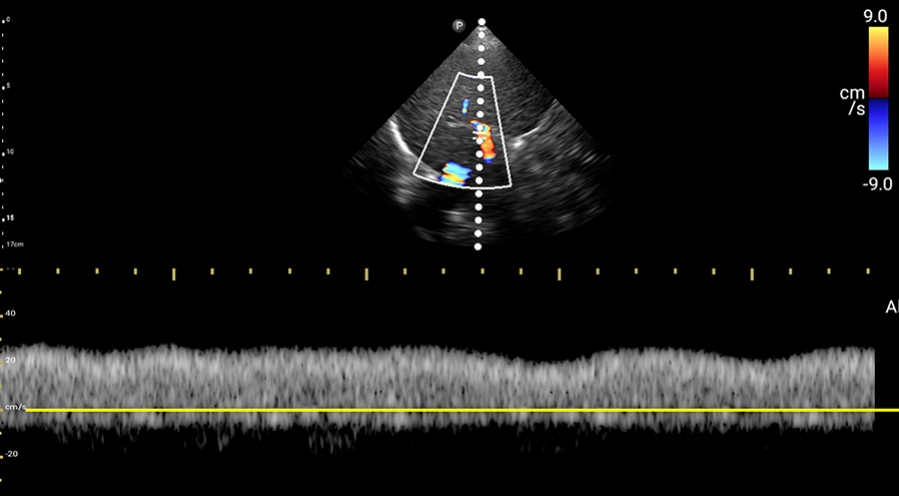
Pulsed-wave Doppler tracing of the PV without hepatic arterial flow interference. PV, portal vein
